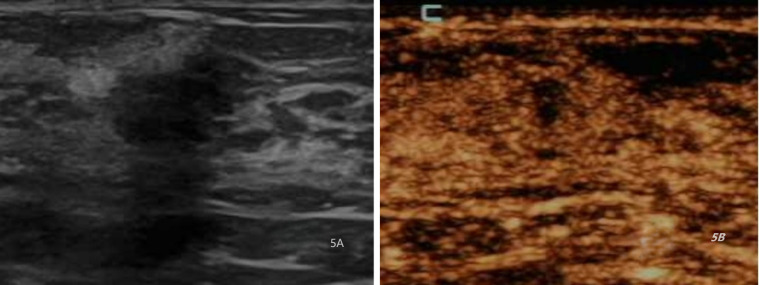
Ultrasound and CEUS images of a 29-year-old female patient with adenosis. (A). Ultrasound (US) image indicating a hypoechoic lesion with unclear borders and irregular morphology., resulting in a total score of 8. (B) Contrast-enhanced ultrasound (CEUS) image depicting homogeneous equal renhancement, indistinct enhancement borders, and the lesion develops a focal perfusion defect, resulting in a total score of 6.
